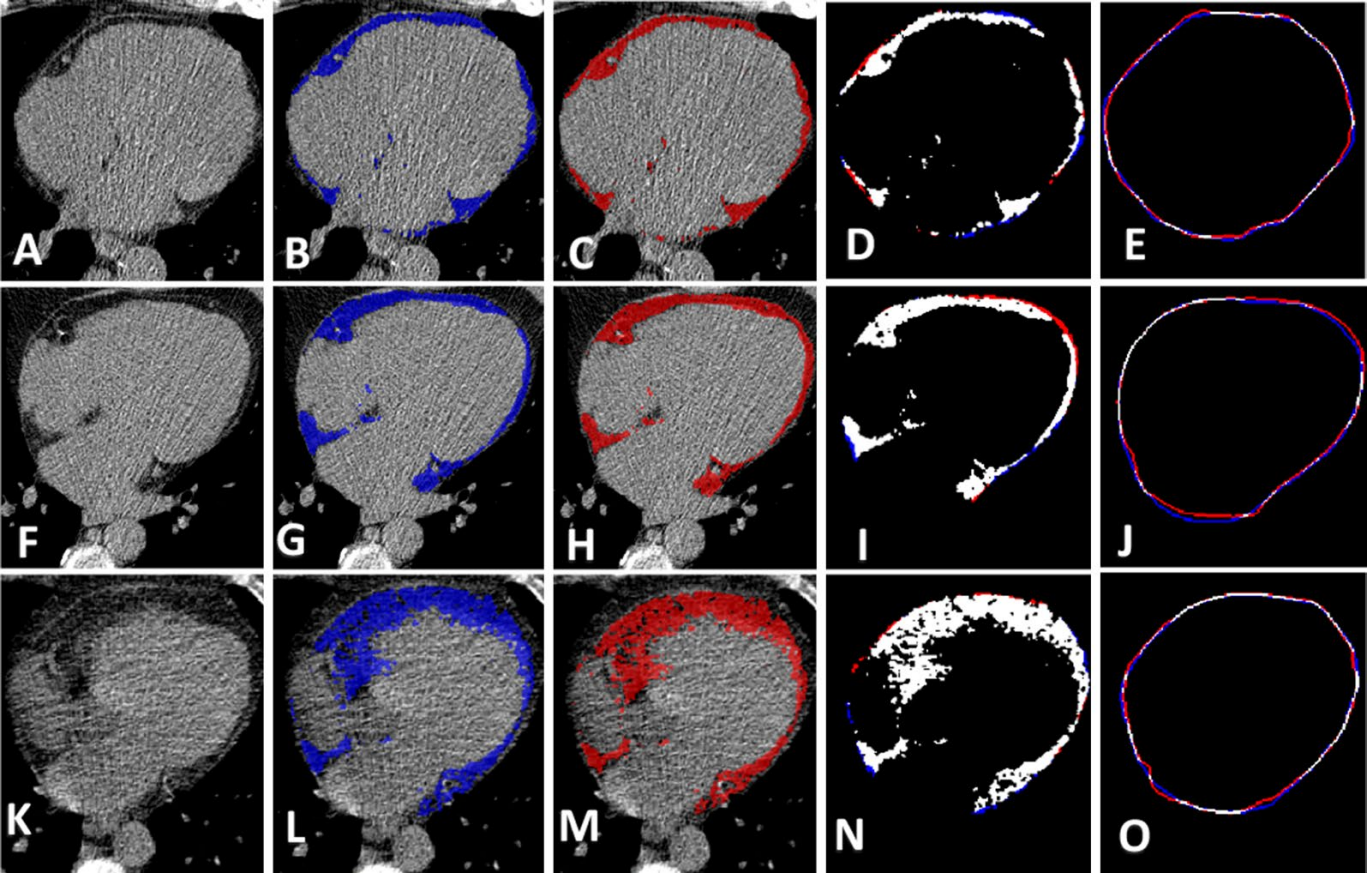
Automated segmentation of epicardial adipose tissue (EAT). Axial non-contrast CT images (A,F,K), manual segmentation in blue (B,G,L), and automated segmentation (using DeepFat with the bisect method) in red (C,H,M). Combined manual and automated EAT segmentation is shown in (D,I,N), where red represents manual, blue represents automatic, and white is the overlapping area. Pericardial sac contours using the same color scheme are shown in (E,J,O). The total subject EAT Dice score is 86.8%, 92.3%, and 92.4%, in the rows with low (A–E), intermediate (F–J), and high fat (K–O). Errors tend to be at the edges of the pericardial sac.
